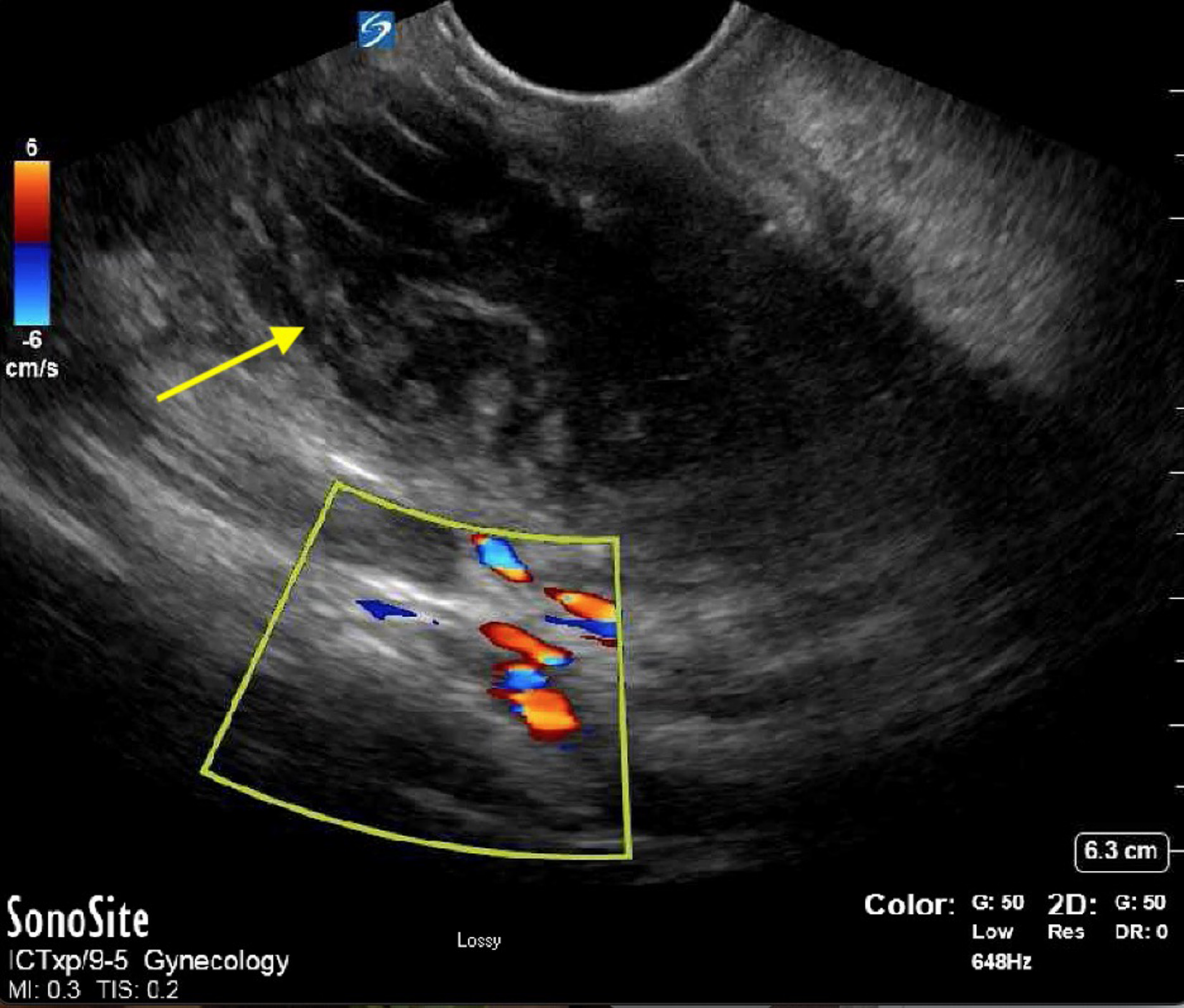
Point-of-care ultrasound with endocavitary probe demonstrating swirling echogenic debris encapsulated approximately 3 centimeters deep, representing initial abscess (arrow). Color box represents vasculature, including carotid artery.
